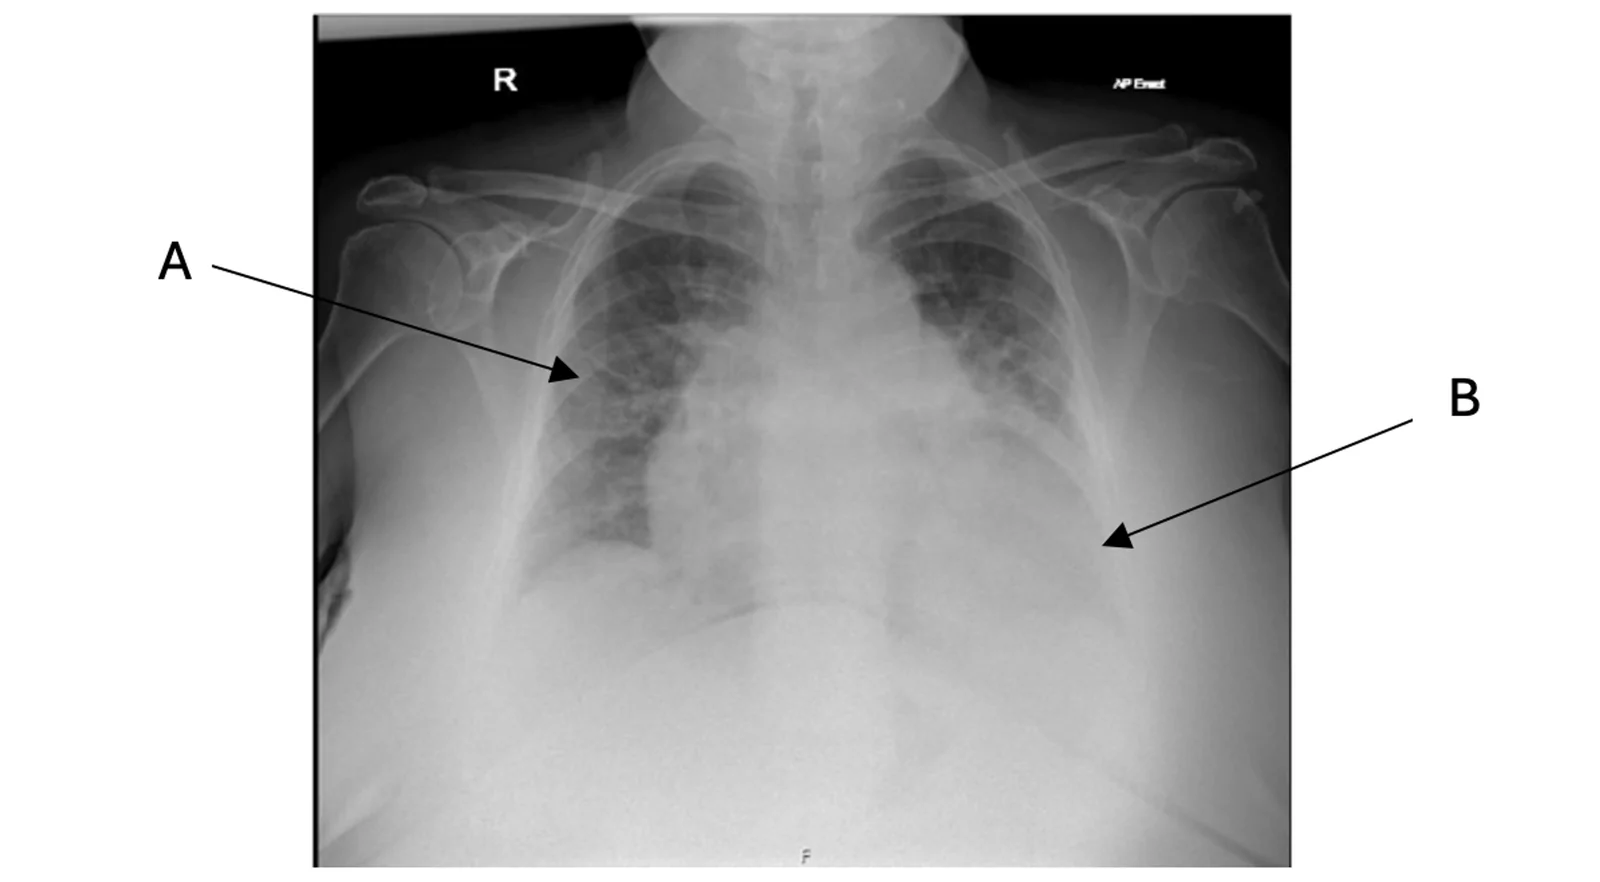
Anteroposterior Chest Radiograph in the Emergency Department Anteroposterior chest radiograph showing bilateral perihilar interstitial and alveolar opacities consistent with pulmonary oedema (labelled A), with associated cardiomegaly, and bilateral pleural effusions (labelled B).
